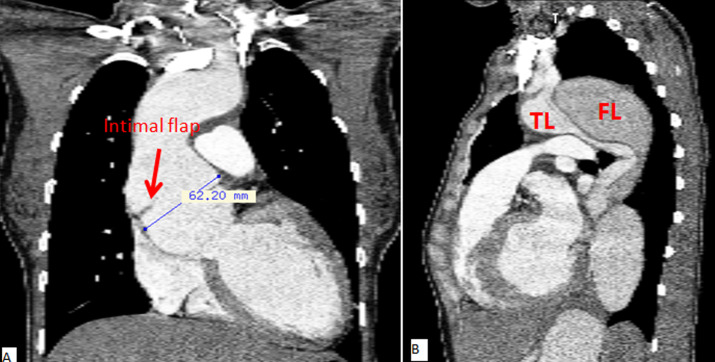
A,B) preoperative computed tomography angiography
